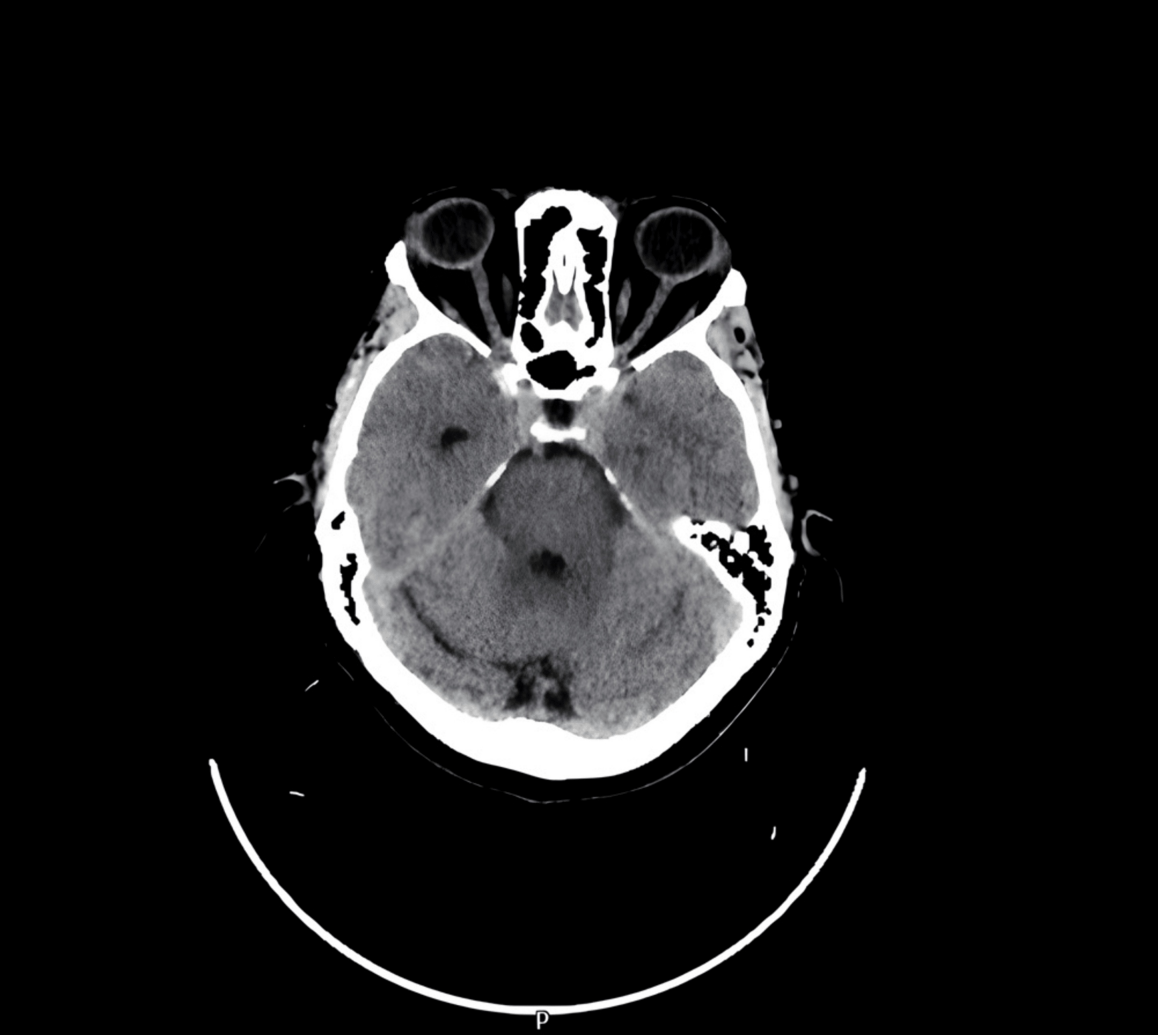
Non-contrast CT head: no acute findings. The petrous bone is intact.
